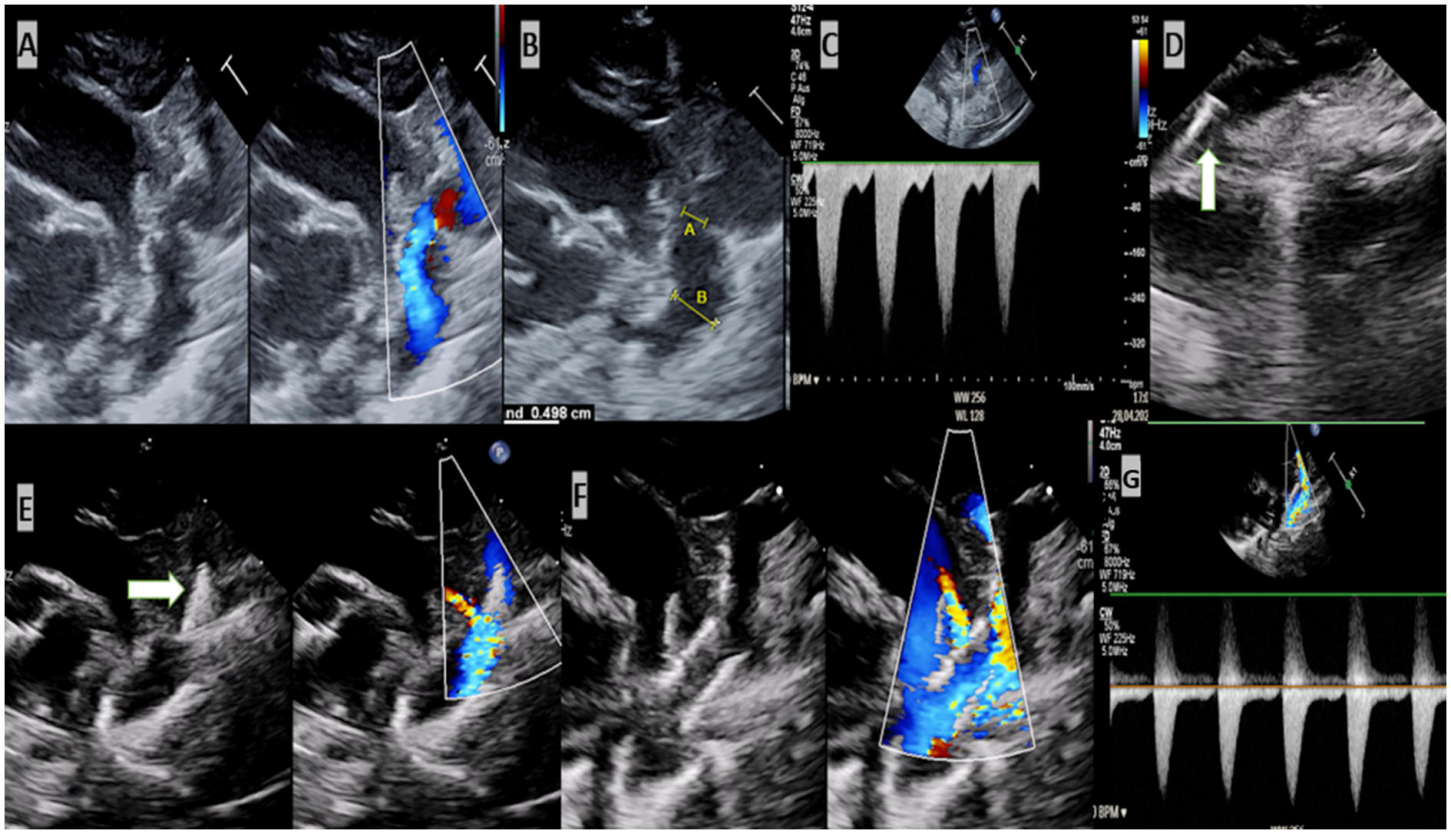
Echocardiogram-guided stenting of critical aortic coarctation. (A) Critical coarctation of aorta. (B) Measurement required for intervention [(A) the diameter of the stenosis and (B) is the diameter of the descending aorta]. (C) Increased velocity of blood in the region of stenosis. Insertion of the wire in the descending aorta. (D) Stent was positioned in the isthmus. (E) Stent was implanted. (F,G) Normal velocity of blood after implantation of stent.
